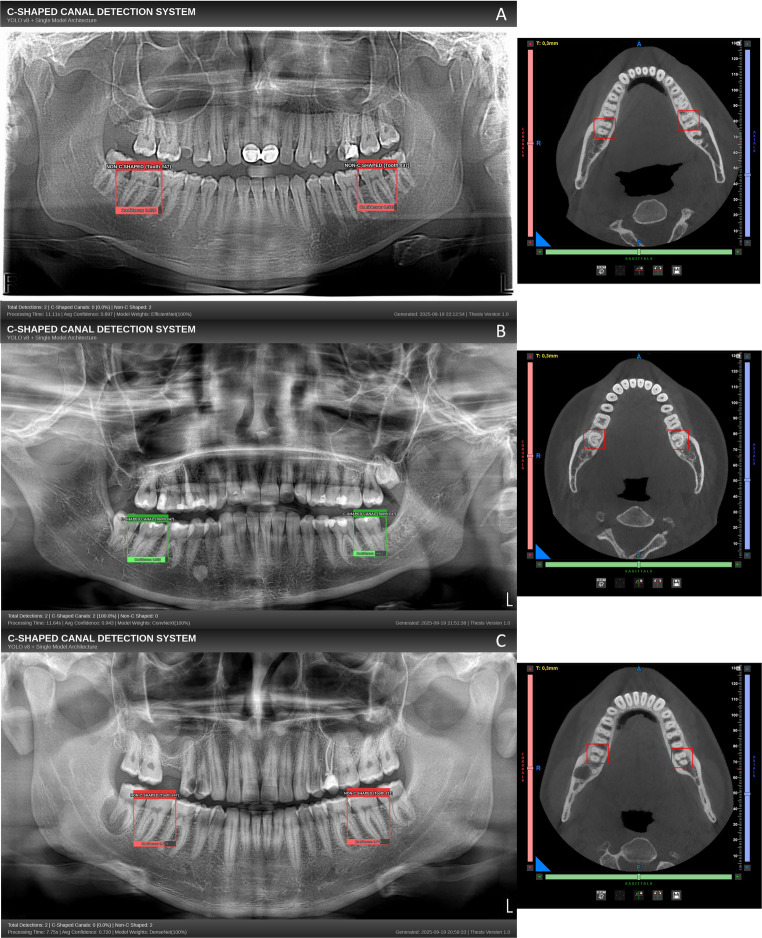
Model outputs on panoramic radiographs (left) and the corresponding CBCT horizontal slices used as the reference standard (right). (A) EfficientNet-B6, (B) ConvNeXt-Base, and (C) DenseNet-169 classifiers. On the panoramic images, green boxes indicate the presence of a C-shaped canal and red boxes indicate its absence; all boxes reflect YOLOv8-based tooth localization. On the CBCT panels, the red boxes delineate the mandibular second molars (#37 and #47) used for ground-truth adjudication
